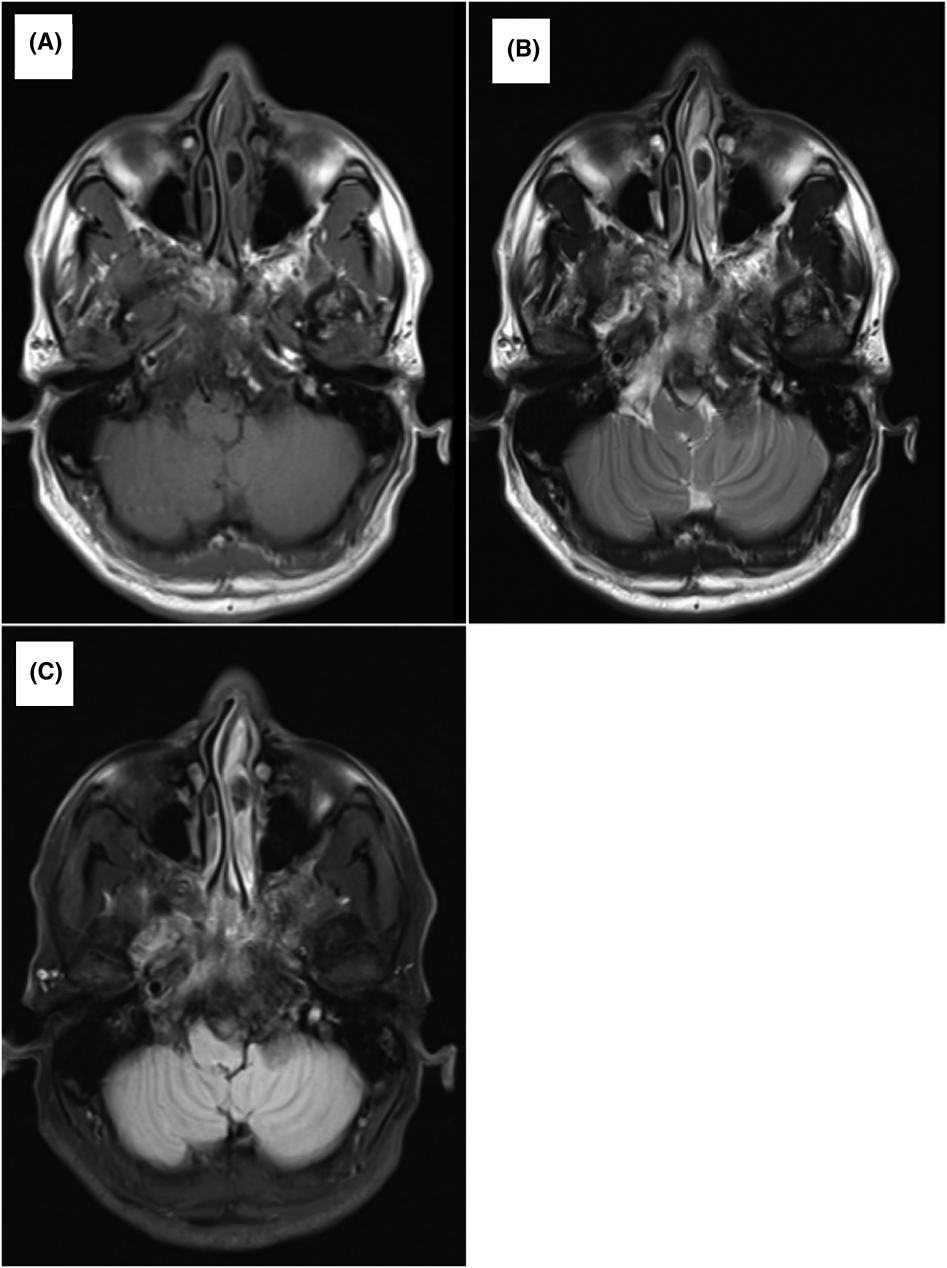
Magnetic resonance image (MRI) brain revealed a destructive skull base lesion eroding the body of sphenoid, clivus mainly inclined toward the right side down to the jugular fossa and occipital condyle with soft mass extending to prepontine, seller, parasellar, and right petrous bone. (A): T1 pre‐contrast; (B) T2 post‐contrast; (C) FLAIR.
